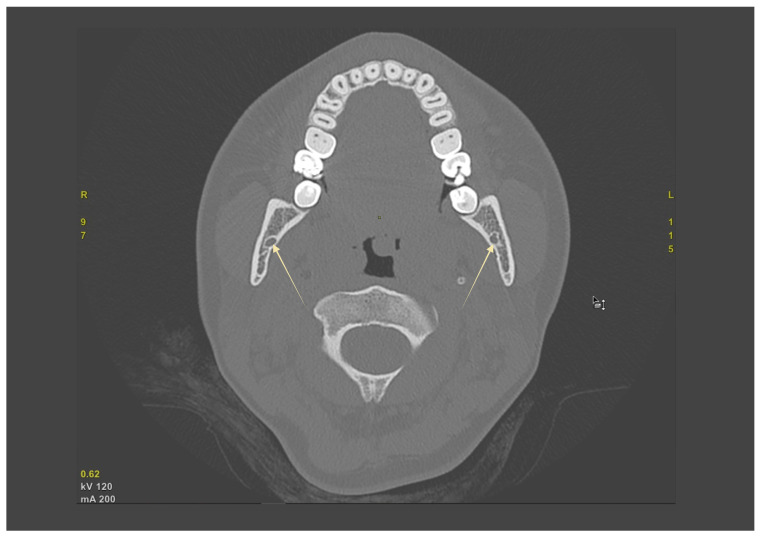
Axial CBCT demonstrating the mandibular canal (MC) bilaterally. Corticated cross-sections of the mandibular canals (yellow arrows) are visible within the mandibular bodies on both sides, allowing direct assessment of canal morphology and symmetry.
